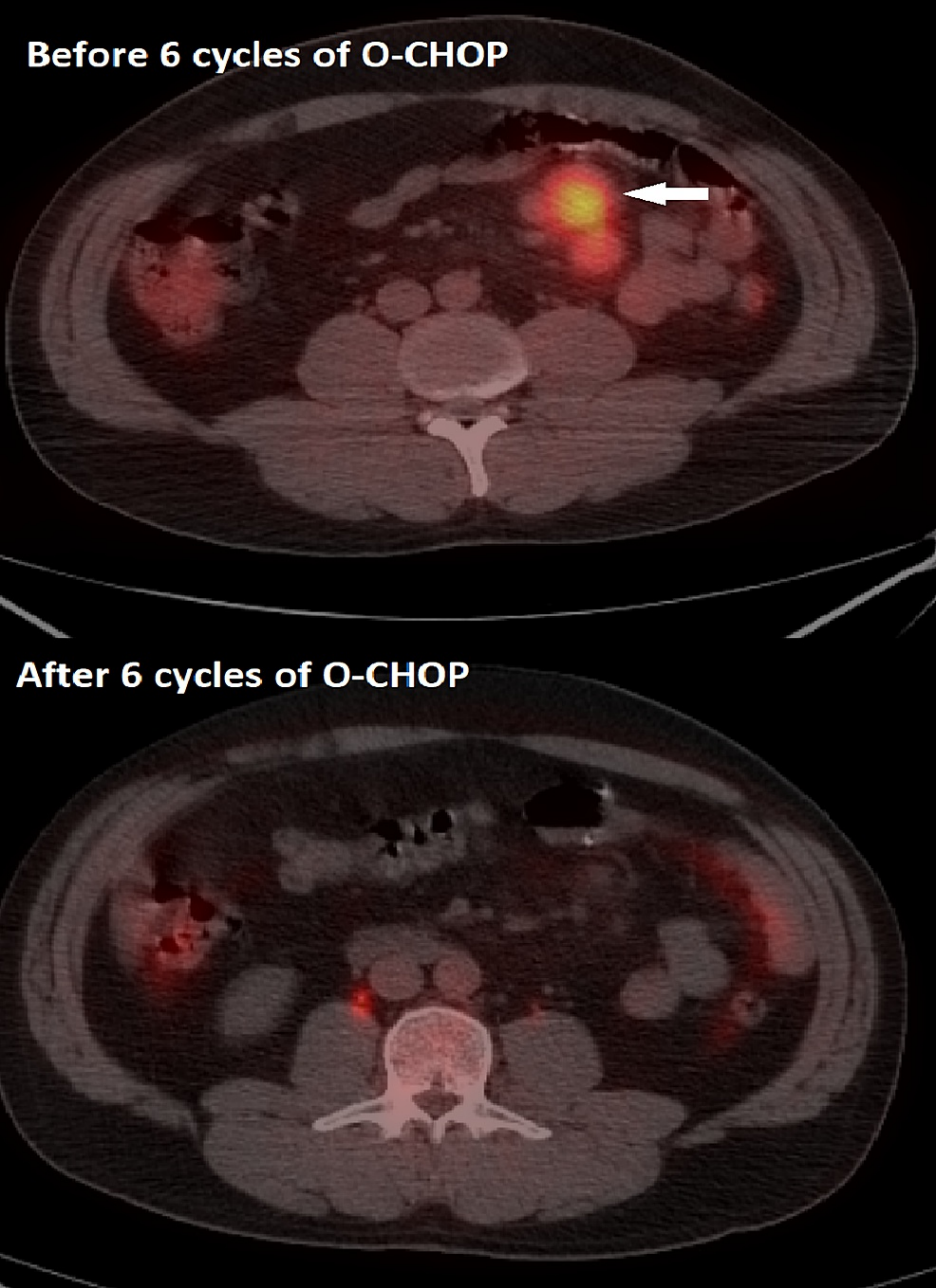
PET/CT scan before and after finishing six cycles of O-CHOP, showing resolution of the hypermetabolic mesenteric lymphadenopathy consistent with complete response to therapy.
